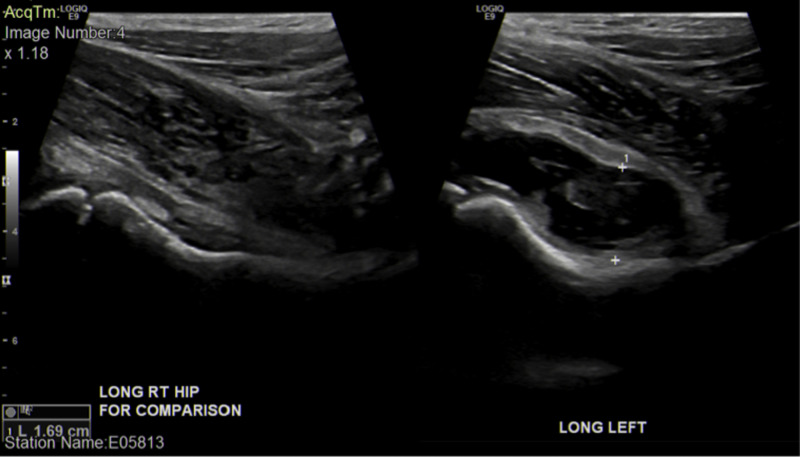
Single long view of the patient’s hips via ultrasound preoperatively. A normal right hip makes the 1.69-cm effusion shown on the left hip more evident. Surrounding hyperemia and loculated effusion versus visualized mass are also seen.
